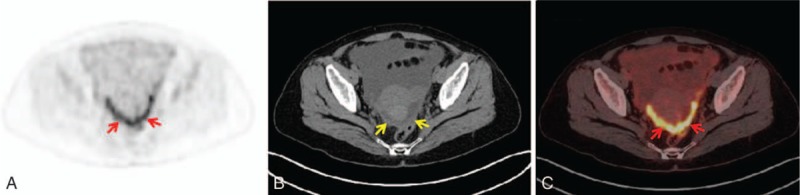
A 51-year-old woman with peritoneal carcinomatosis of gastric cancer: clustered ^18^F-FDG uptake in the parietal peritoneum (red arrow) on PET and combined PET/CT axial imaging (A and C); irregular thickening in the pelvic region (yellow arrow) on CT axial imaging (B). CT = computed tomography,^ 18^F-FDG = F18-fluorodeoxyglucose, PET = positron emission tomography.
